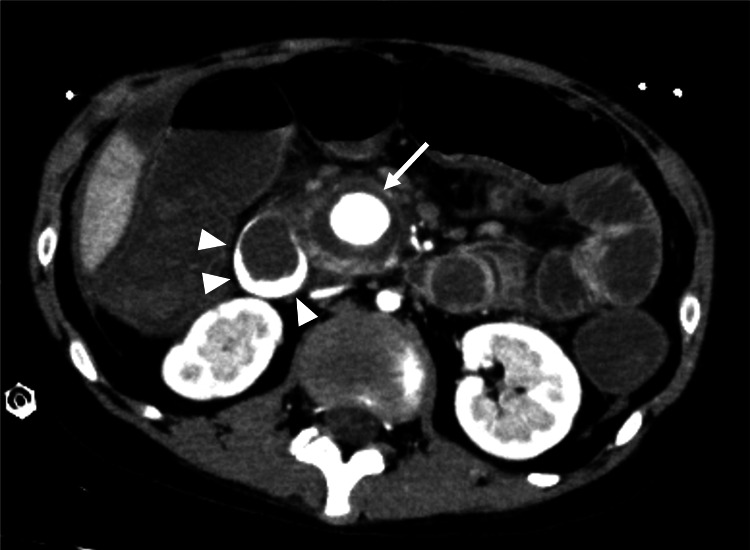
Contrast-enhanced computed tomography shows a massively enlarged pseudoaneurysm (white arrow) surrounded by a hematoma Contrast medium leaked into the duodenum (arrowheads)
